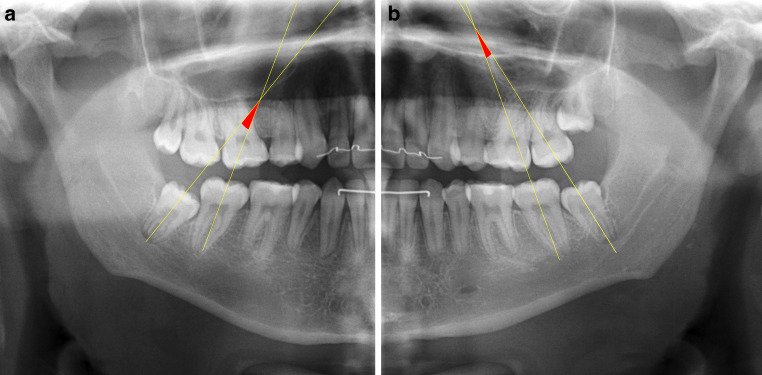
Lines used to measure the third molar angulation on the orthopantomograms. Measured angulations of +16.19° for the patient’s right third molar (a) and +8.65° for the patient’s left third molar (b) Zur Messung der Angulation des dritten Molaren auf den Orthopantomogrammen verwendete Linien. Gemessene Winkel von +16,19° für den rechten dritten Molaren des Patienten (a) und +8,65° für den linken dritten Molaren des Patienten (b)
